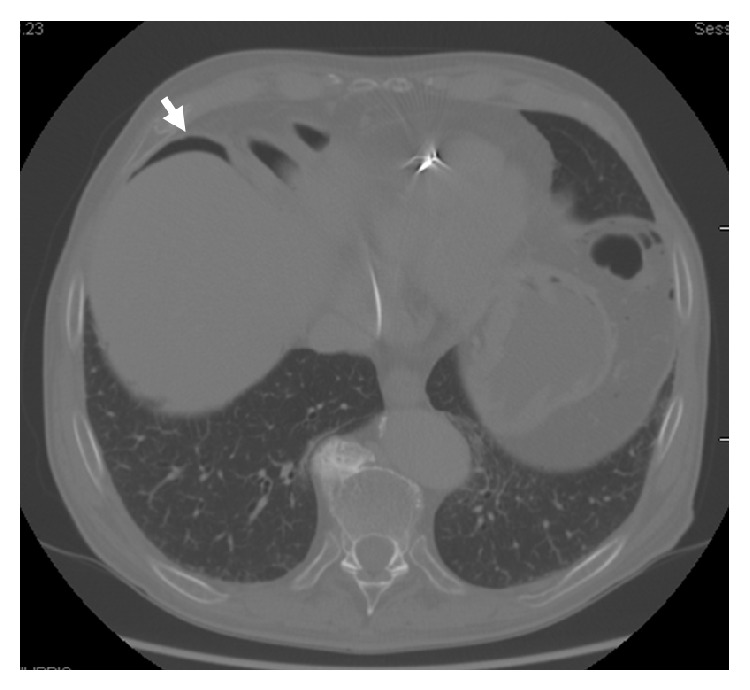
Abdominal CT scan showing free intraperitoneal air near the liver.
